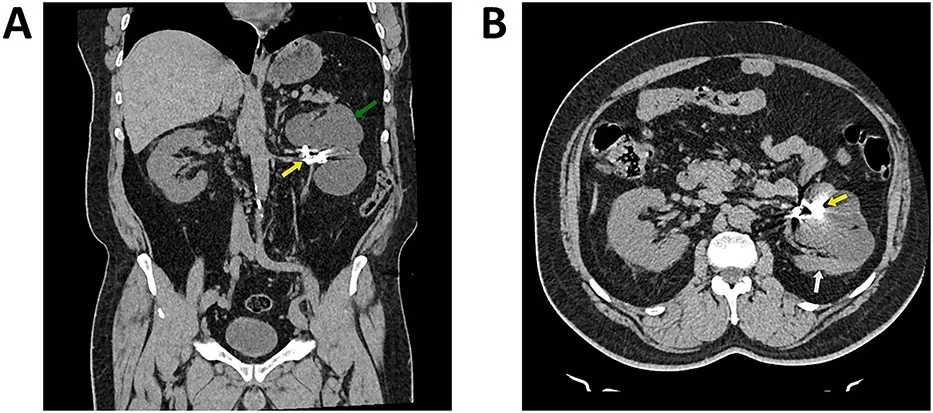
Native abdominal CT. A. Coronal section. B. Axial section. It shows severe hydronephrosis of the left kidney (green arrow), a small part of parenchyma is remaining posteriorly (white arrow), other parts show marked renal parenchymal loss, the cause is a metallic foreign body impacted in the UPJ and renal pelvis.
